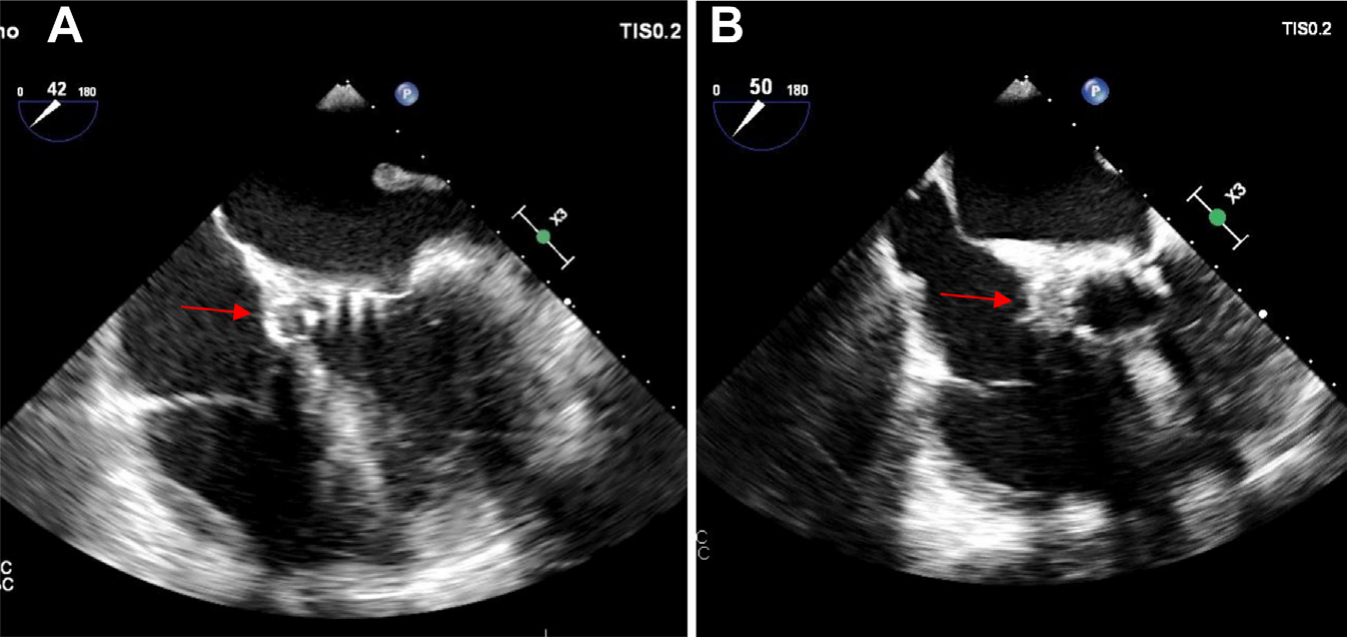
Transesophageal Echocardiography Images (A) A 4-chamber view showing prosthetic aortic valve (acoustic shadow of the valve struts seen) with perivalvular oval opacity with hypoechoic centre. (B) A more short axis view of the prosthetic valve with para valvular opacity (red arrow indicating the paravalvular lesion).
